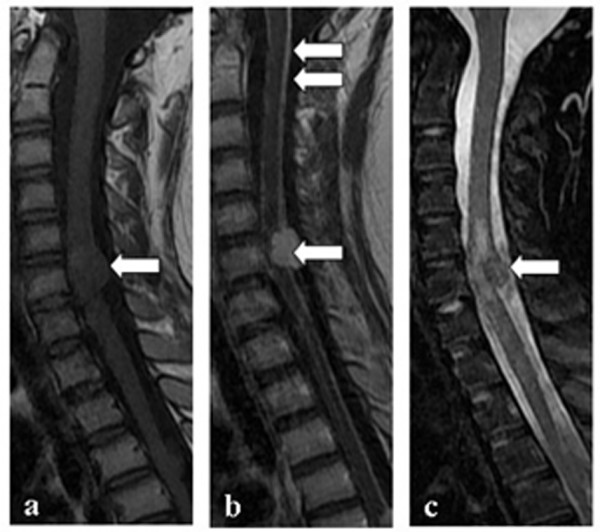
Spinal MRI findings in a CNS-TB patient with spinal cord involvement. a: tuberculoma with iso-intensity on sagittal T1-weighted image; b: T1-weighted contrast image showing enhancement of the whole lesion and meninges; c: tuberculoma with iso-intensity on a sagittal T2-weighted image.
